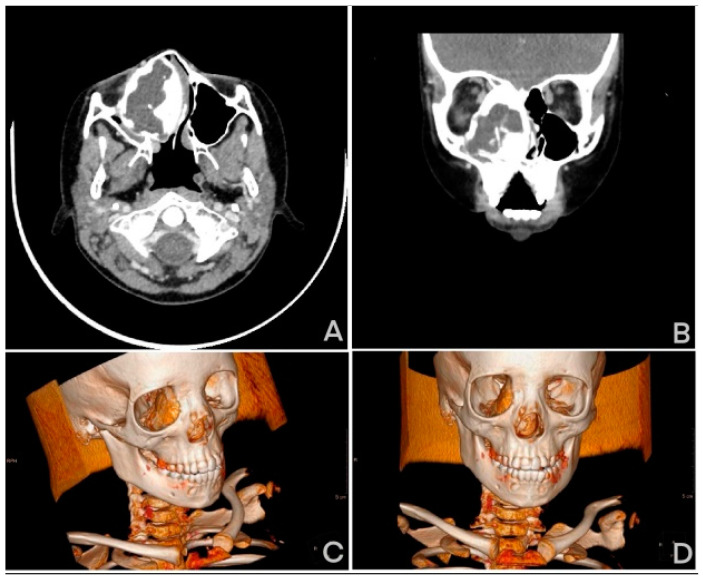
CT image in: (A) sagittal projection, (B) frontal, (C,D) 3D image reconstructions.
